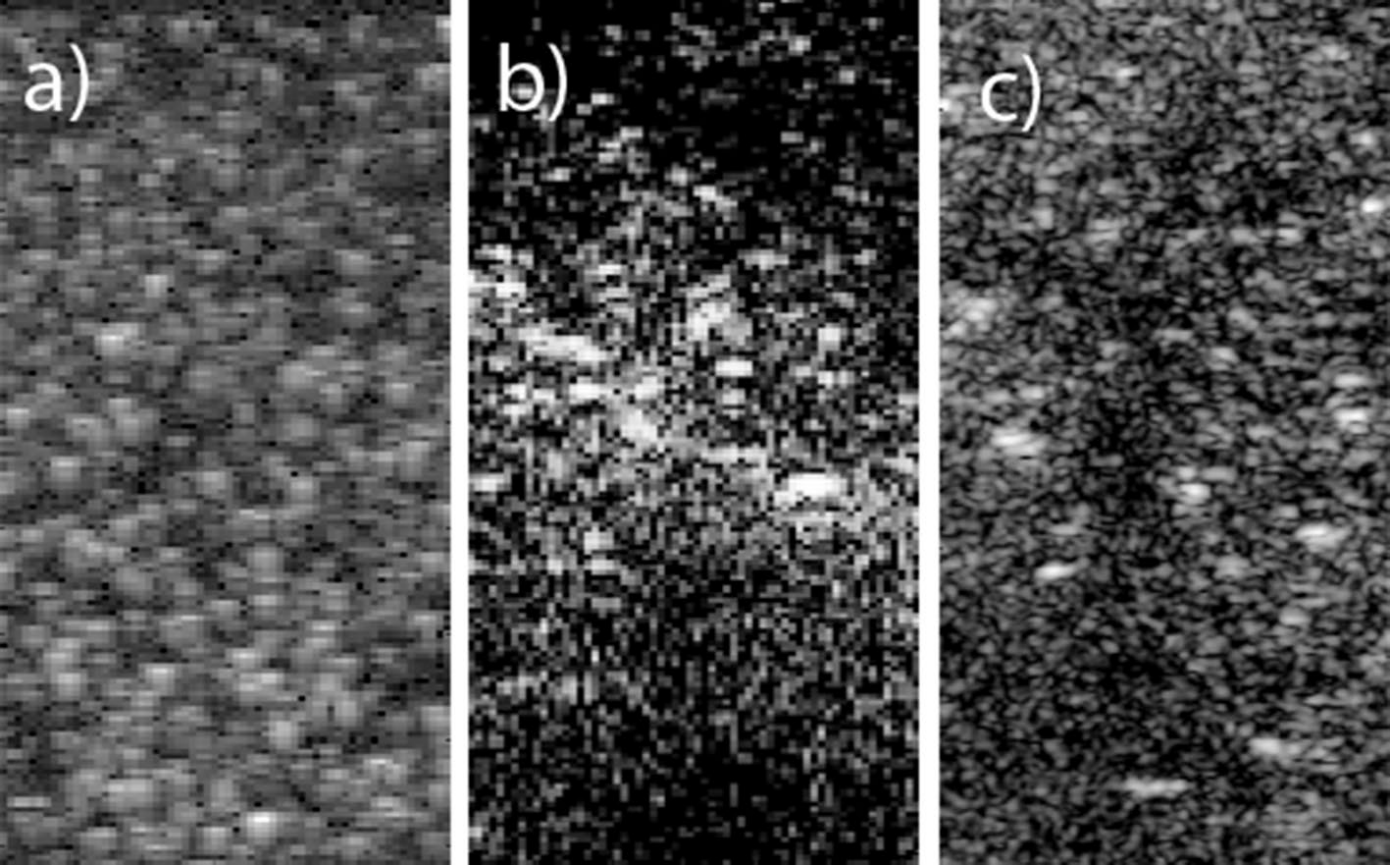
B-mode images from the three ultrasound sources: a in silico, b ULAOP, and c Philips EPIQ 7. The images each depict an area of 20 × 15 mm^2^
